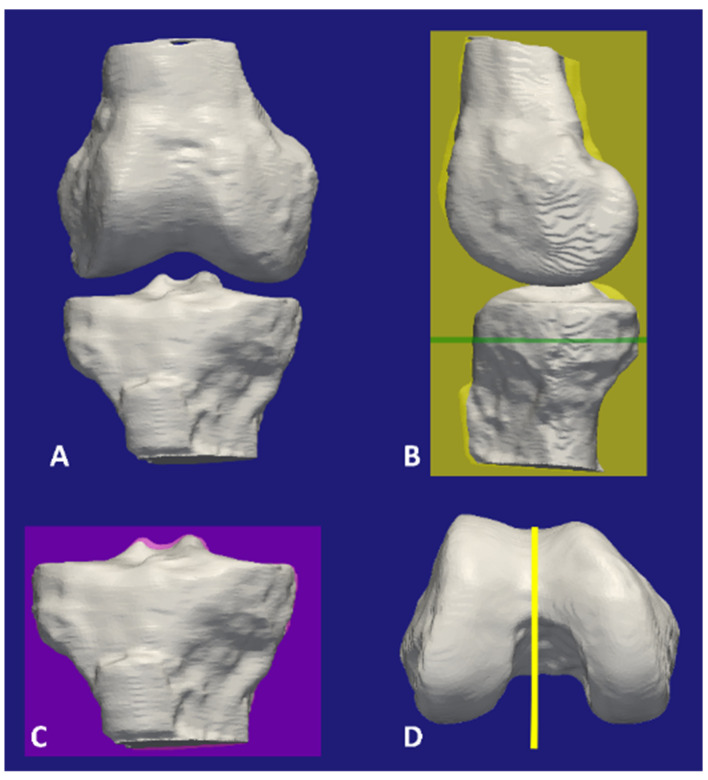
Images showing a 3D model of an extended right knee (A) and the steps for orientating the knee in the standard planes (B–D). The knee model was developed by registering the 3D models of the tibia and femur generated from laser scanning with the respective bones in the 3D model of the intact knee from the first CT scan (A). The standard sagittal plane (yellow) was formed by superimposing the posterior femoral condyles [5,6,7,8,9], the standard axial plane (green) was normal to the standard sagittal plane and parallel to the medial surface of the tibial plateau (B), and the standard coronal plane (purple) was mutually perpendicular to the other two standard planes (C). The line on the distal femur was parallel to the standard sagittal plane (D).
